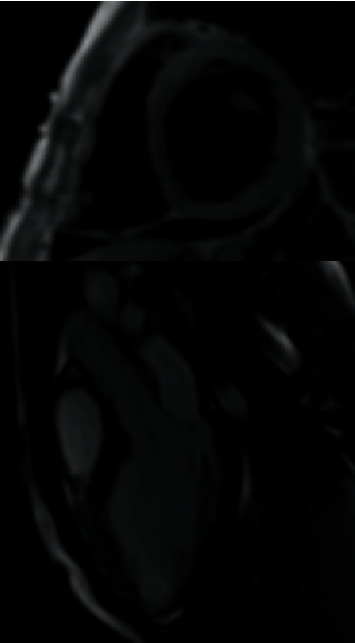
Cardiac MRI showed mildly dilated LV.
